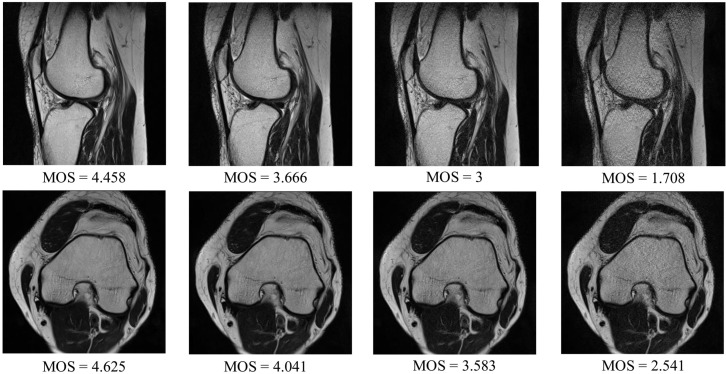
Introduced MRIQA benchmark (DB2): Exemplary MR images and their subjective scores.
